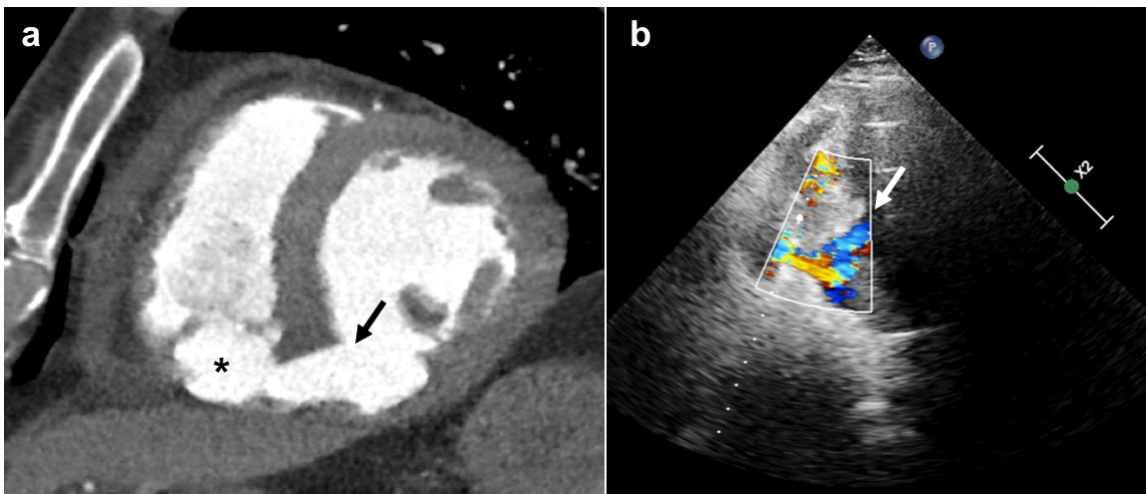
Imaging demonstration of VSD. (a) CTTA biventricular short axis view showing an inferior and inferoseptal LV wall defect of mid-ventricular cavity (black arrow) and an oval cavity included in the RV (black asterisk). (b) TTE shows the LV to RV shunt through the LV wall interruption (white arrow). CCTA, cardiac CT angiography; LV, left ventricle; RV, right ventricle; TTV, transthoracic echocardiography; VSD, ventricular septal defect.
